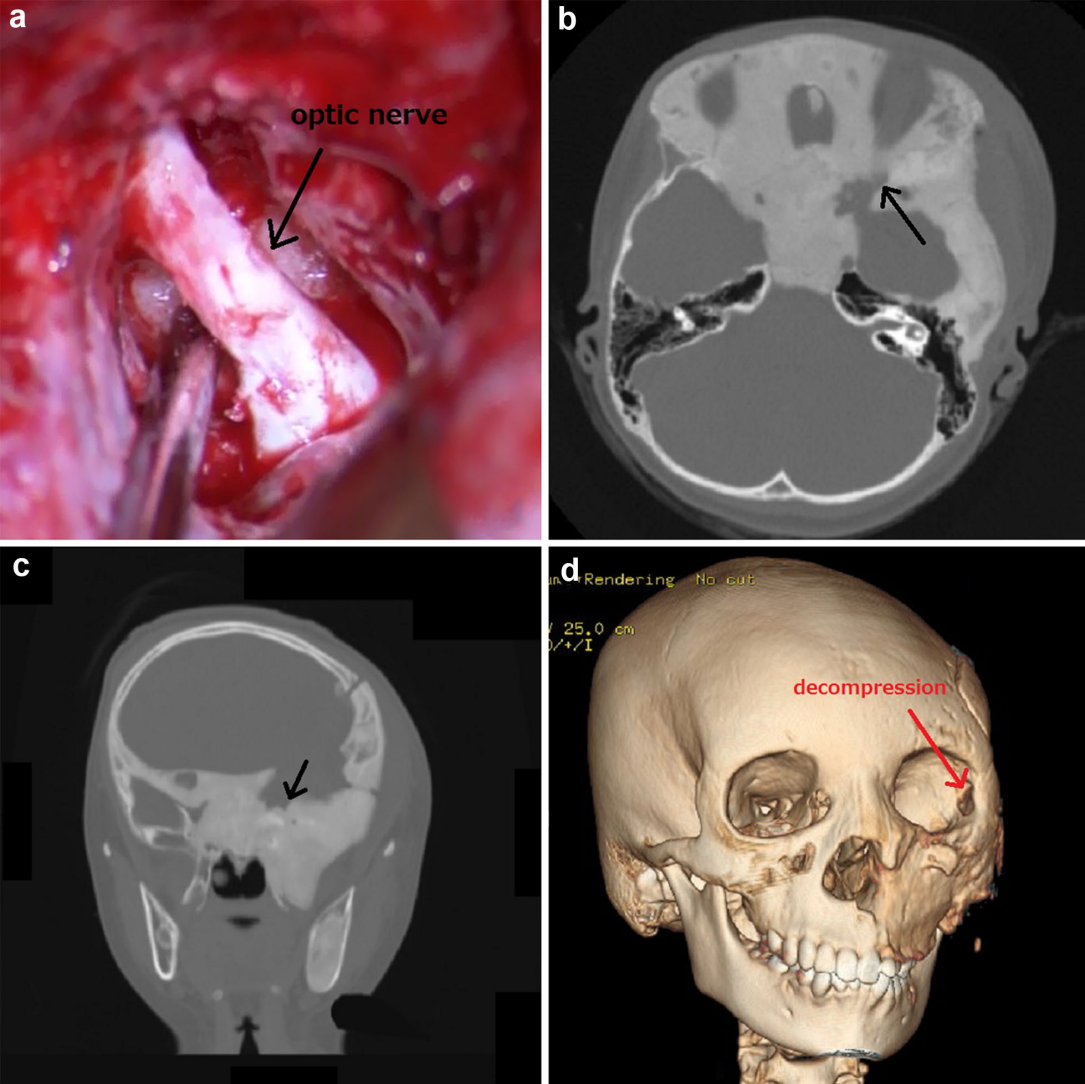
a The optic nerve is sufficiently decompressed via epidural decompression. b, c High-resolution head axial and coronal CT shows sufficient decompression of the optic canal (*arrows*, decompressed optic canal). d Bone 3 dimensional-CT shows improvement in the *left* facial bone. In addition, it shows decompression of the lateral orbital wall
